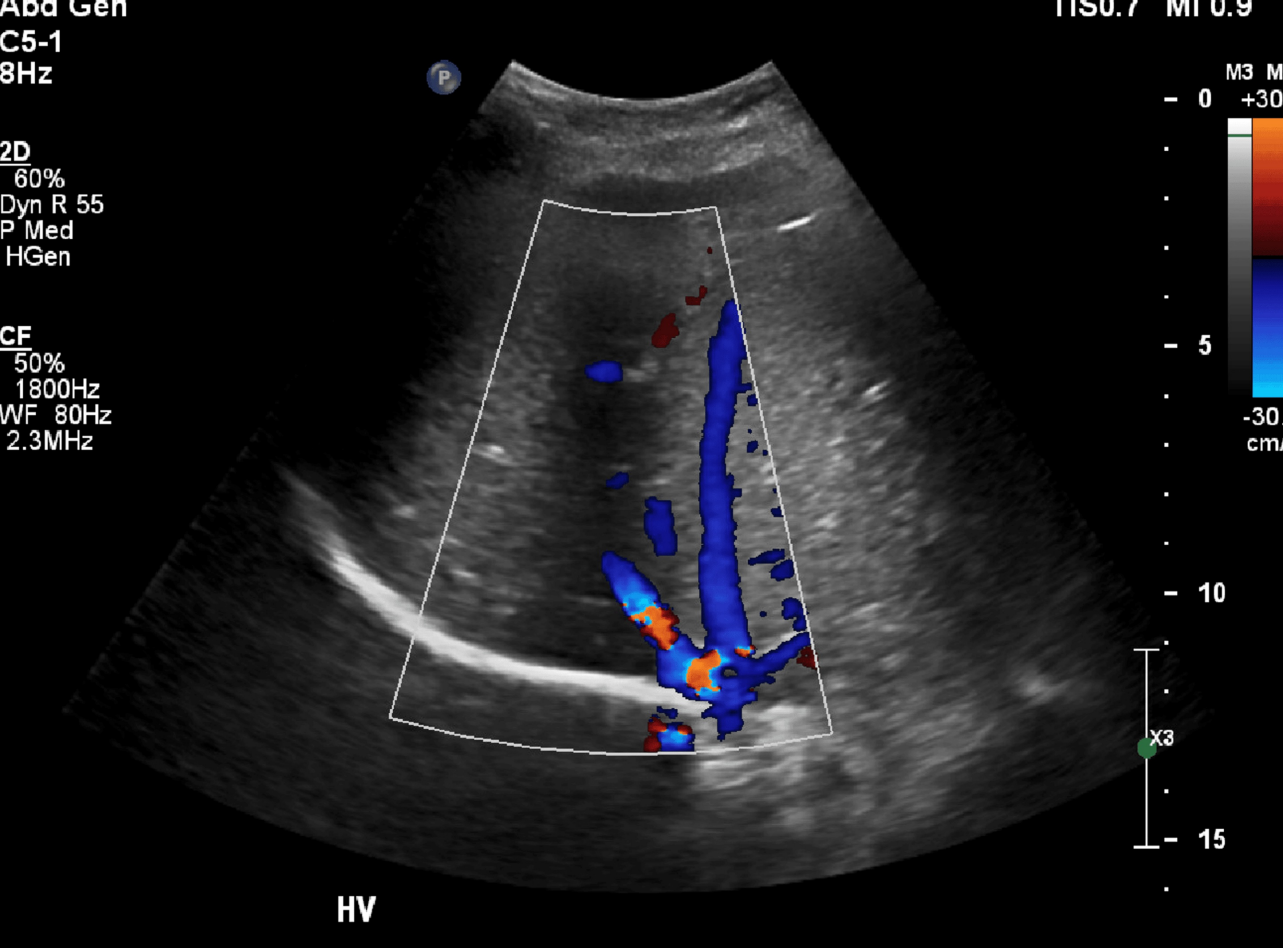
Doppler ultrasound of the liver showing patent portal vein with no filling defect
